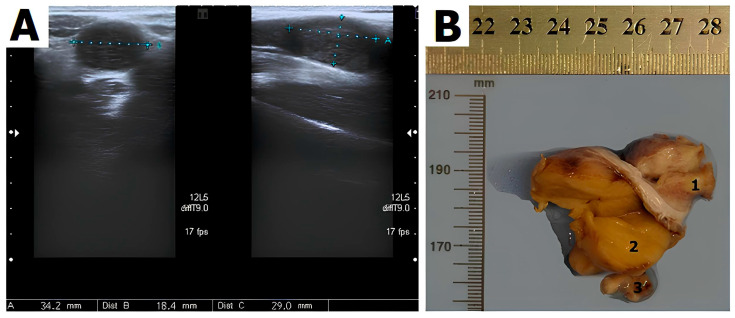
(A) Transvaginal ultrasonography demonstrating the gonads (testes) located medial to iliac vessels. The right gonad measured 31 × 12 × 11 mm, the left gonad 34 × 18 × 21 with medium-level echogenicity. (B) Gross examination of the left gonad: leiomyoma (1), Sertoli–Leydig tumor (2), fallopian tube (3) (previously unpublished original photos).
